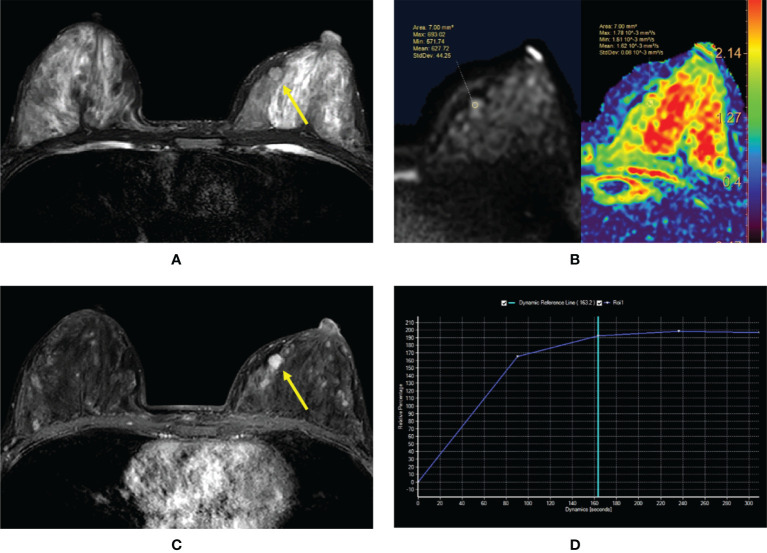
(A–D) Intraductal papilloma in a 38-year-old woman. (A) T2-weighted image showing an isointensity signal mass lesion (yellow arrow) in the left breast. (B) Diffusion-weighted imaging showing a hyperintensity signal and apparent diffusion coefficient (ADC) map showing mean ADC = 1.62 × 10^-3^ mm^2^/s. (C) Enhanced T1-weighted image showing a strong nodular enhancement (yellow arrow) with clear margins. (D) Time–signal intensity curve manifests as a rapid increase (initial phases) and a plateau type (delayed phases).
